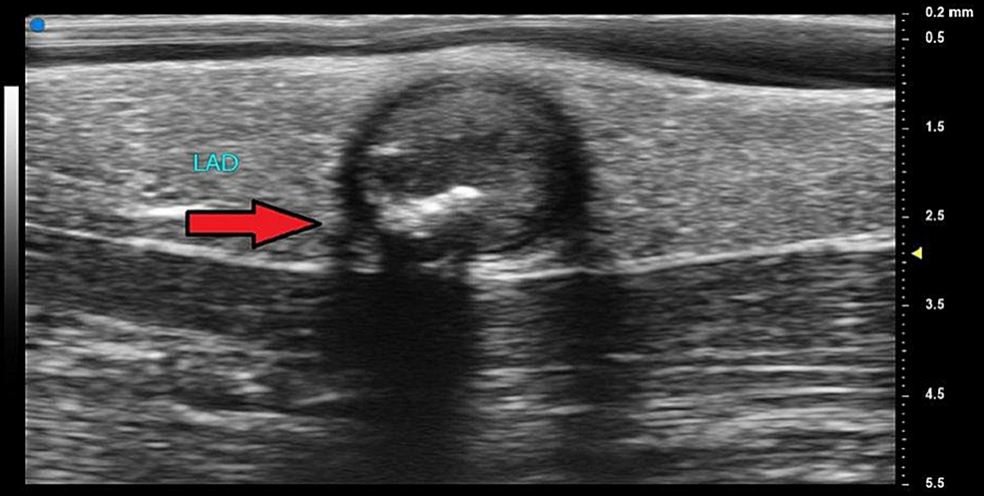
Short axis view of the left anterior descending artery The arrow shows the LAD in short axis, and calcifications are noted for both their bright echogenicity and ultrasonic dropout artifact. LAD - left anterior descending
